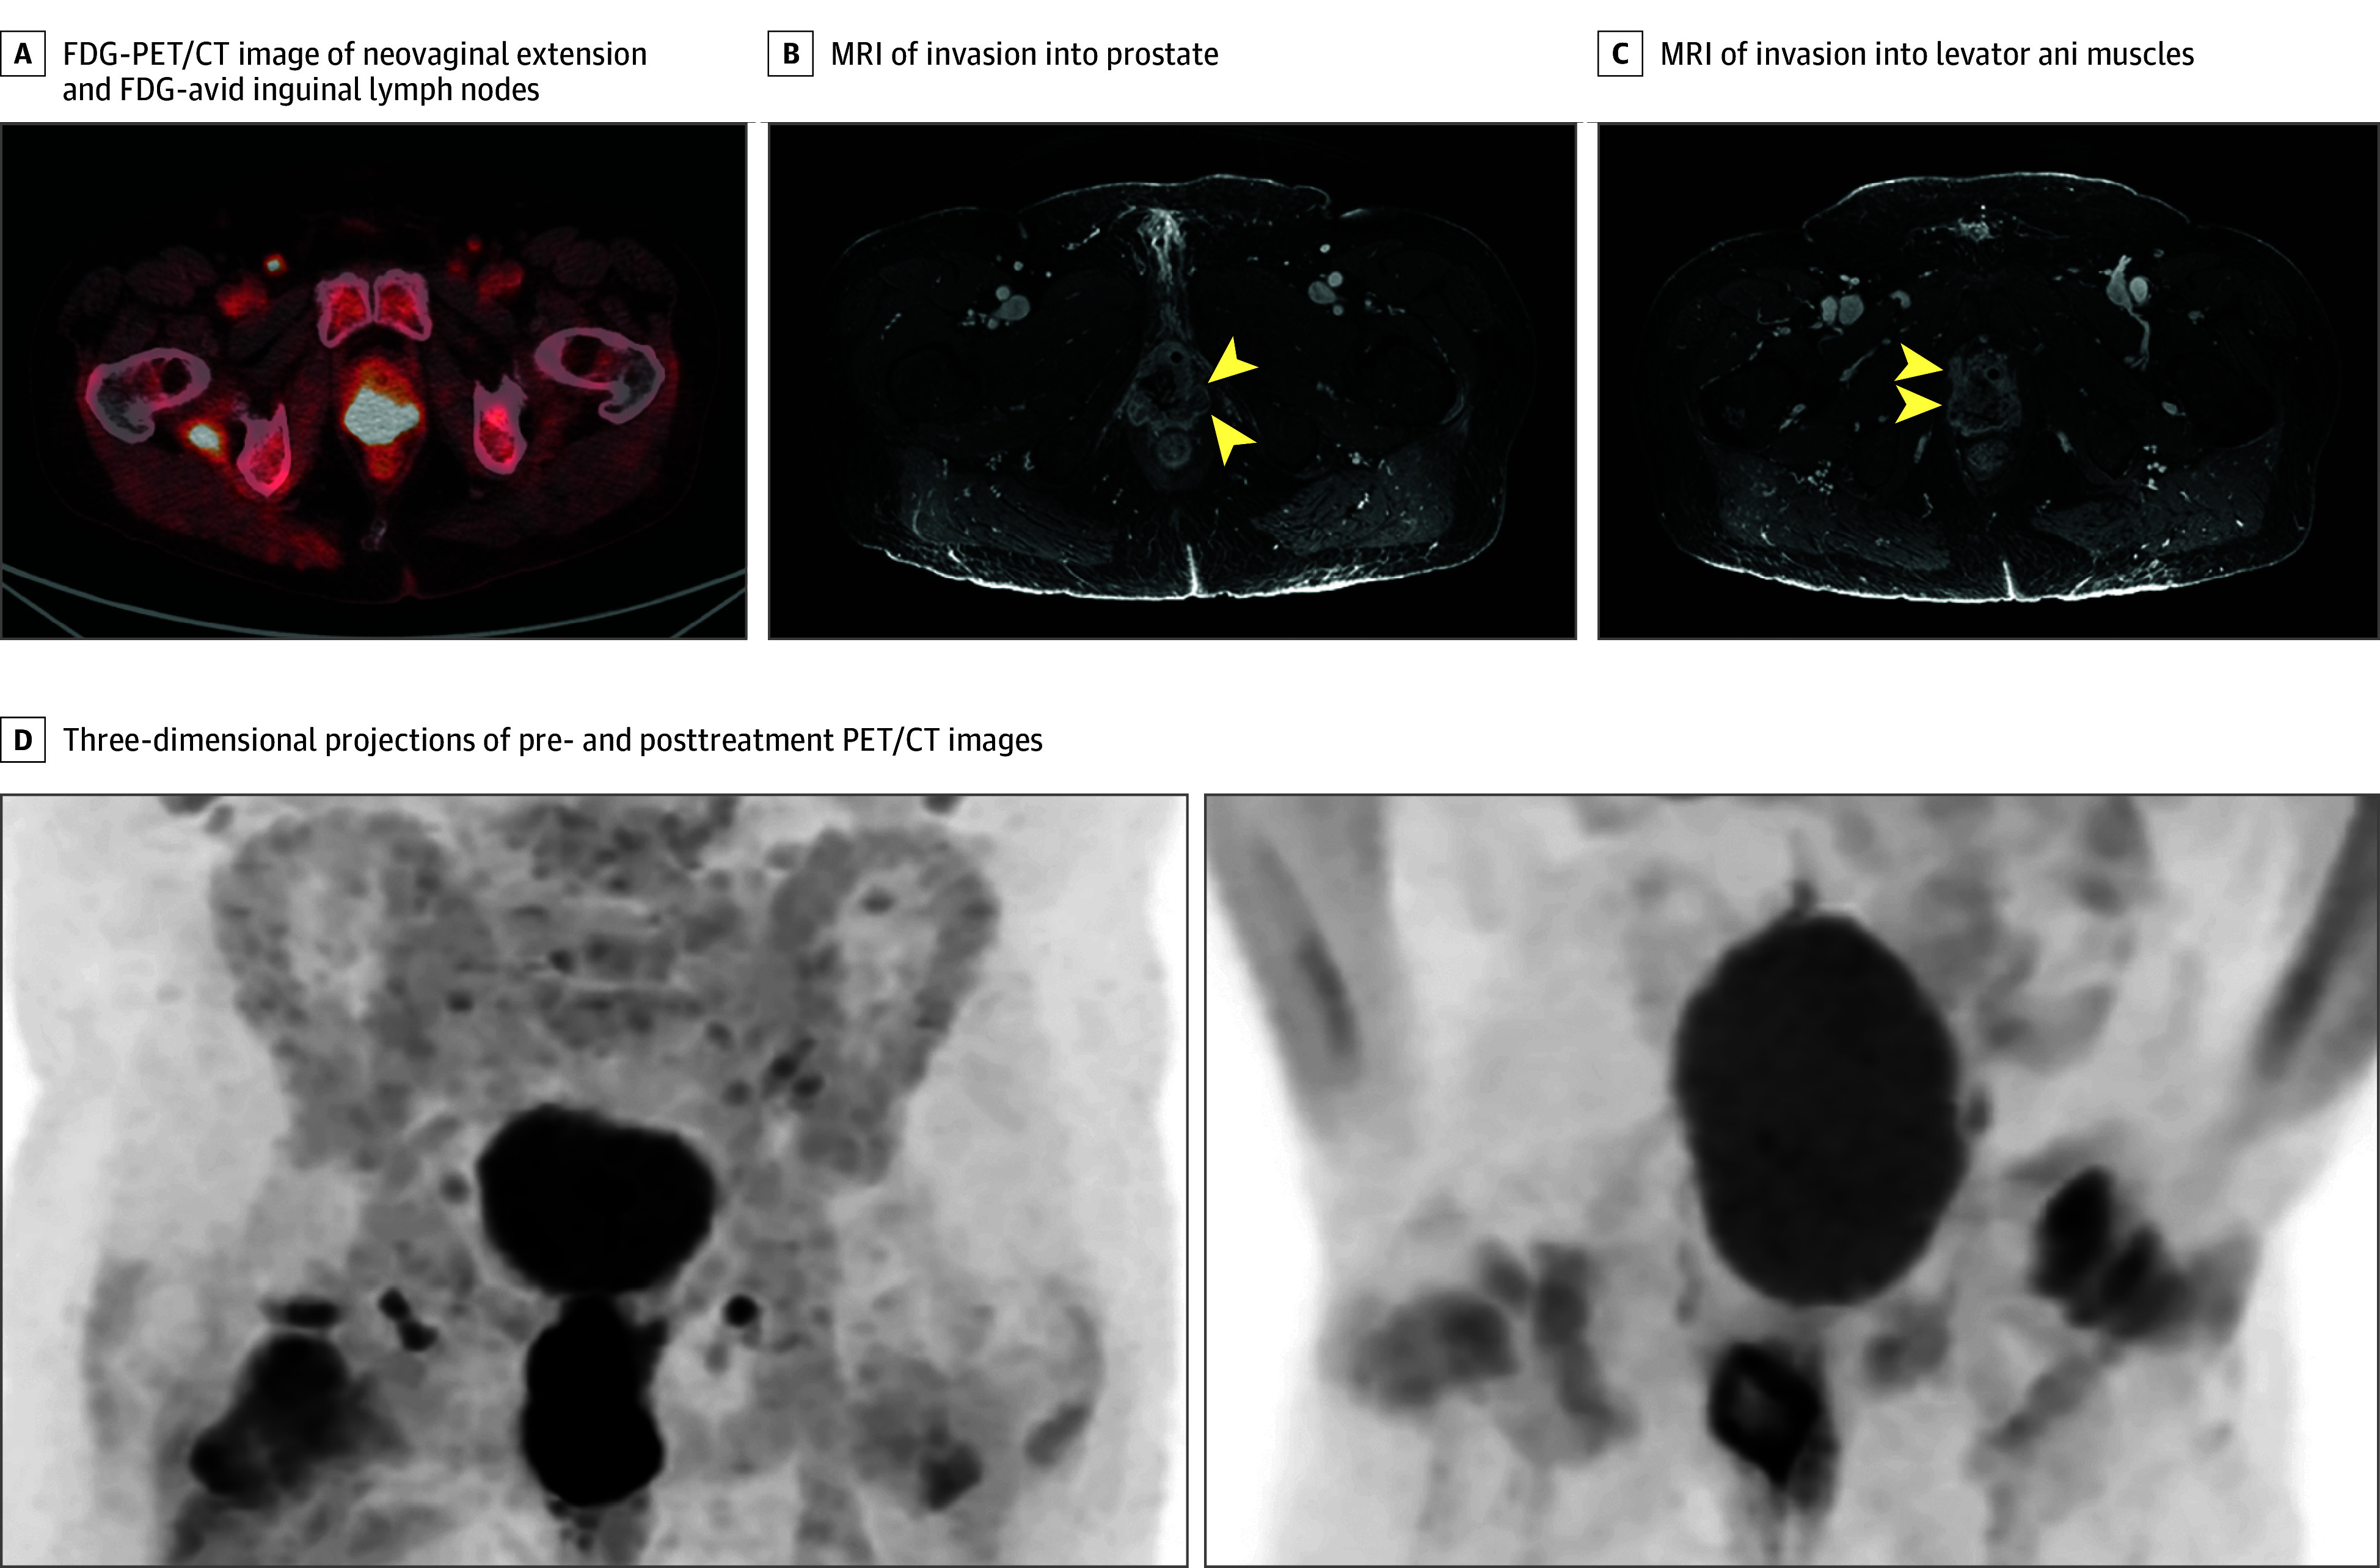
Imaging of Human Papilloma Virus–Related Neovaginal Squamous Cell Carcinoma Extent Arrowheads in panels B and C indicate invasion into the prostate and levator ani bilaterally. FDG-PET/CT indicates fluorodeoxyglucose–positron emission tomography/computed tomography; MRI, magnetic resonance imaging.
